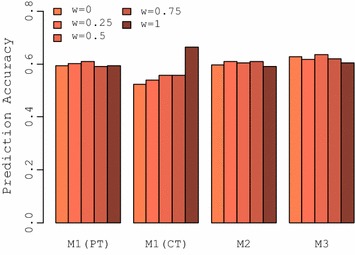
Accuracy of selection for five selection criteria for each genetic model. Accuracies are presented for breed A only. M1 (PT): genetic Model 1 with purebred training, M1 (CT): genetic Model 1 with crossbred training
